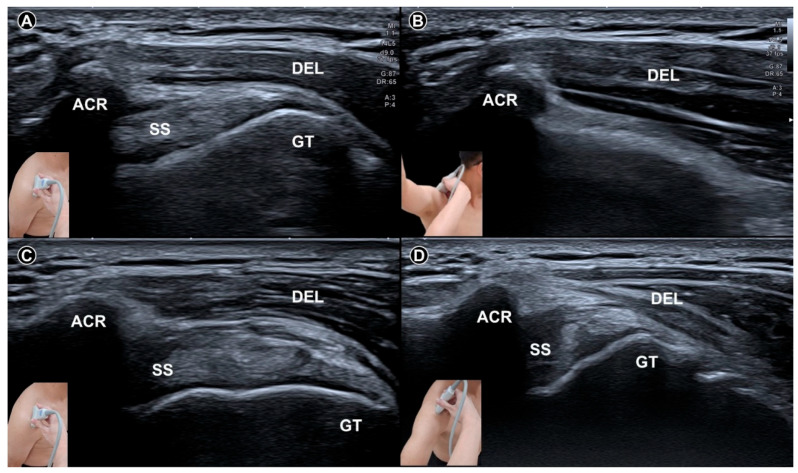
Dynamic ultrasound imaging of the subacromial region at neutral resting position (A) and during shoulder abduction (B) in a shoulder without adhesive capsulitis shows the greater tubercle of the humerus rotating beneath the acromion. In contrast, in a shoulder with adhesive capsulitis, the greater tubercle remains outside the acromion even during maximal shoulder abduction (C) resting; (D) maximal abduction. The figure was redrawn by the authors with reference to Ryu et al. [33]. ACR, acromion; SS, supraspinatus tendon; GT, greater tubercle; DEL, deltoid muscle.
